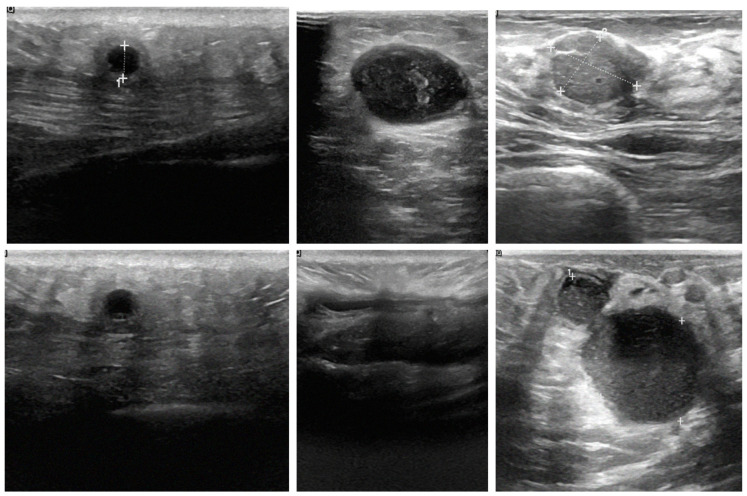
Examples of varying resolutions in breast sonography images.
